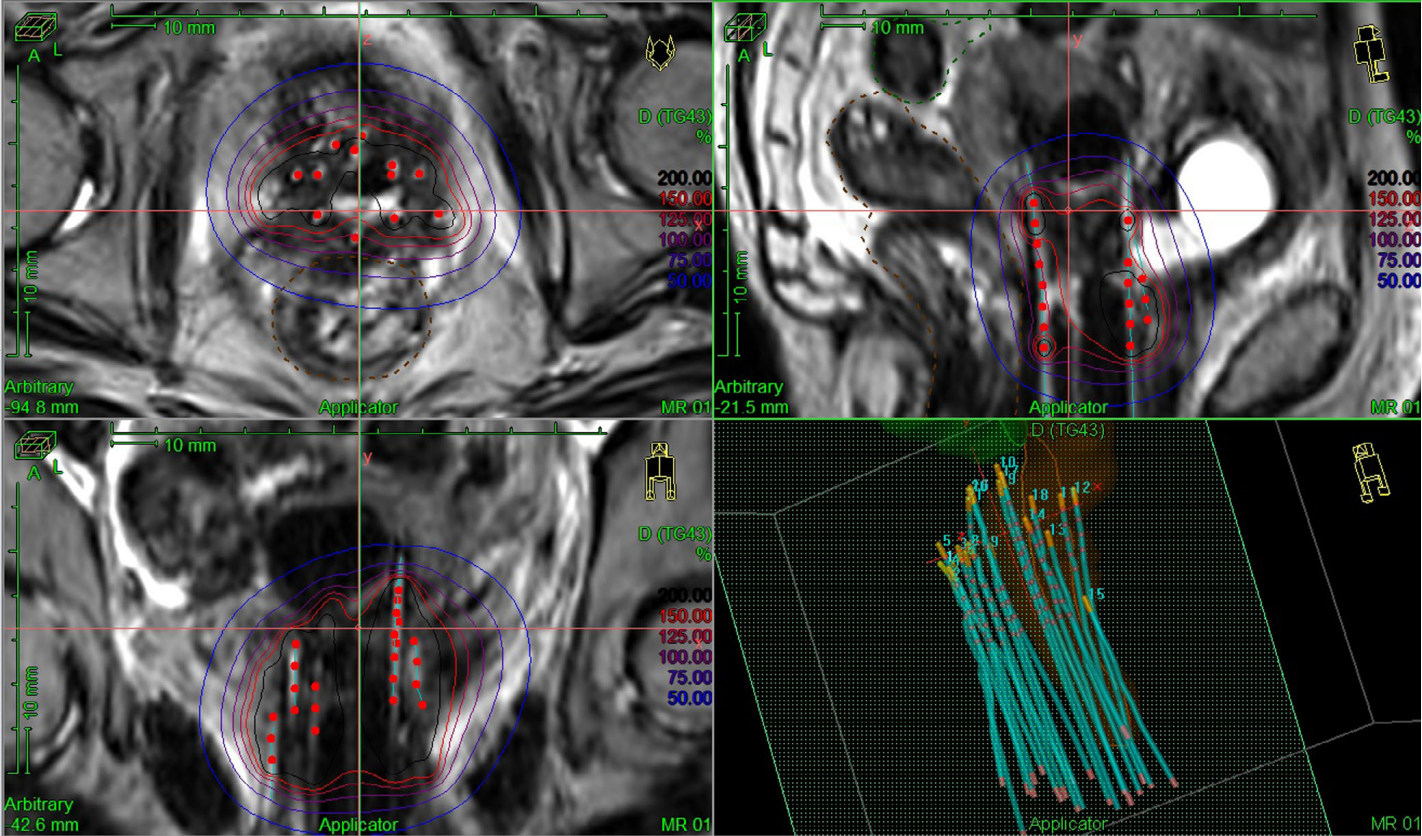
A typical case of MRI‐HDR‐IBT plan seen in a TPS. TPS, treatment planning systems.
